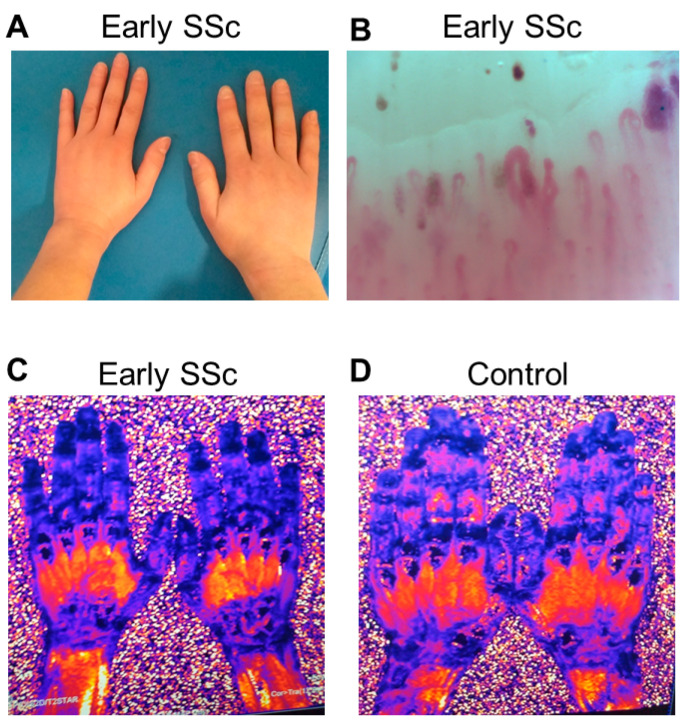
Increased iron levels in the hands of a SSc patient. A woman with early SSc and sclerodactyly (A) underwent capillaroscopy, which revealed multiple nailfold microhemorrhages (arrows) (B). Using a magnetic resonance imaging protocol for evaluation of iron deposition, depicted as black color in the T2(STAR) images, her fingers showed T2(STAR) values of 3 to 3.3 ms (C) denoting iron deposition compared to an apparently healthy control subject with higher values of 9.4 to 11 ms (D).
